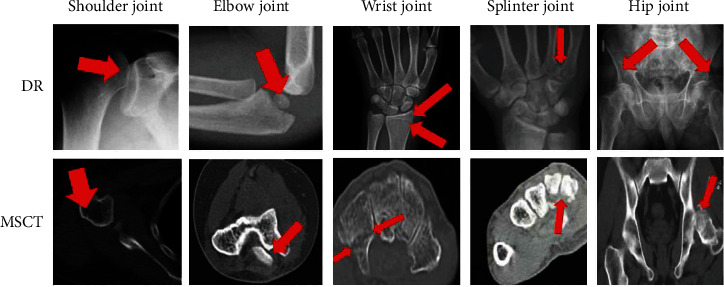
X-ray DR plain film and MSCT image.
